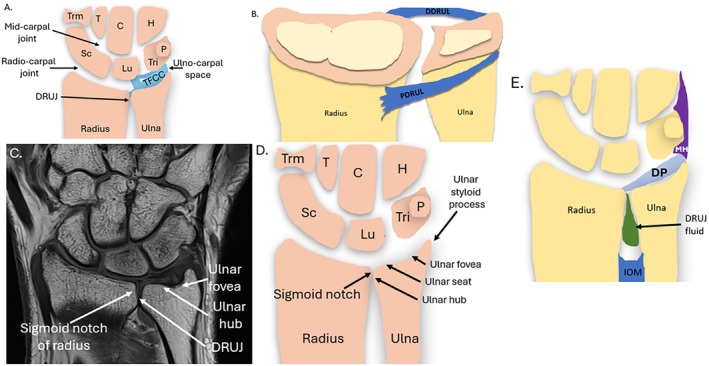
The anatomy of the wrist joints including the distal radio‐ulnar joint (DRUJ) and the ulno‐carpal space. (A) Outline of wrist joints. (B) The palmar and dorsal distal radioulnar ligaments of the DRUJ. (C) Coronal magnetic resonance image of the wrist. (D) Components of the ulnar head. (E) Proximal extension of DRUJ fluid. C, capitate; DDRUL, dorsal distal radio‐ulnar ligament; DP, disc proper; H, hamate; IOM, interosseous membrane; Lu, lunate; MH, meniscus homologue; P, pisiform; PDRUL, palmar distal radio‐ulnar ligament; Sc, scaphoid; T, trapezoid; TFCC, triangular fibrocartilage complex; Tri, triquetrum; Trm, trapezium.
